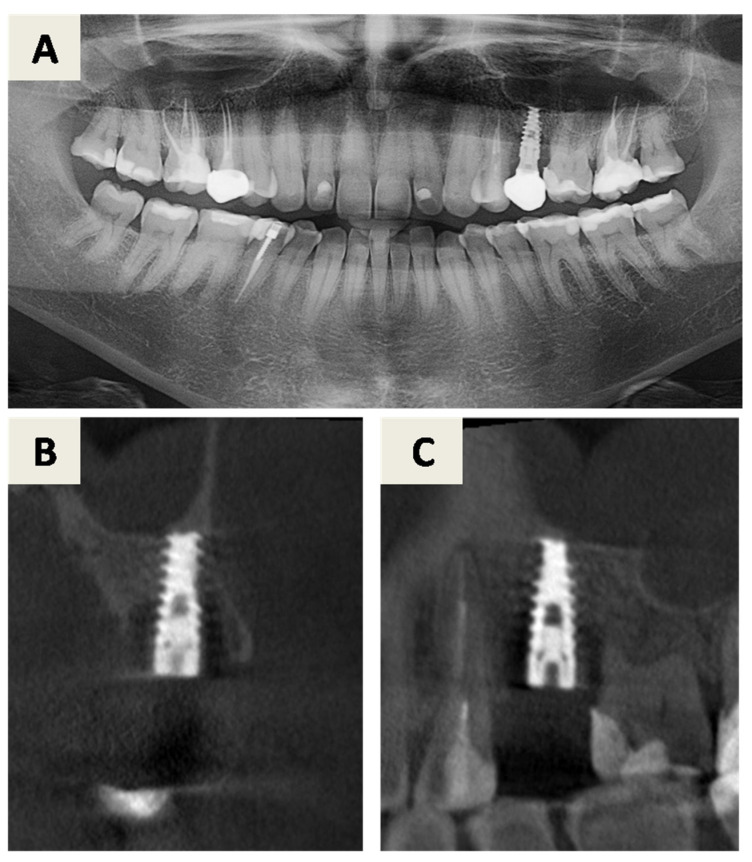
First radiographic examination. (A) Orthopantomogram showing visible peri-implantitis related bone defect around the implant in position 25 (FDI notation system). (B,C) Cone beam computed tomography (CBCT) images in coronal (B) and sagittal (C) planes taken 6 weeks after crown removal showing visible defect of the palatal bony surface extending to ½ length of the implant and a small (2–3 mm) supracrestal defect located around the implant shoulder.
